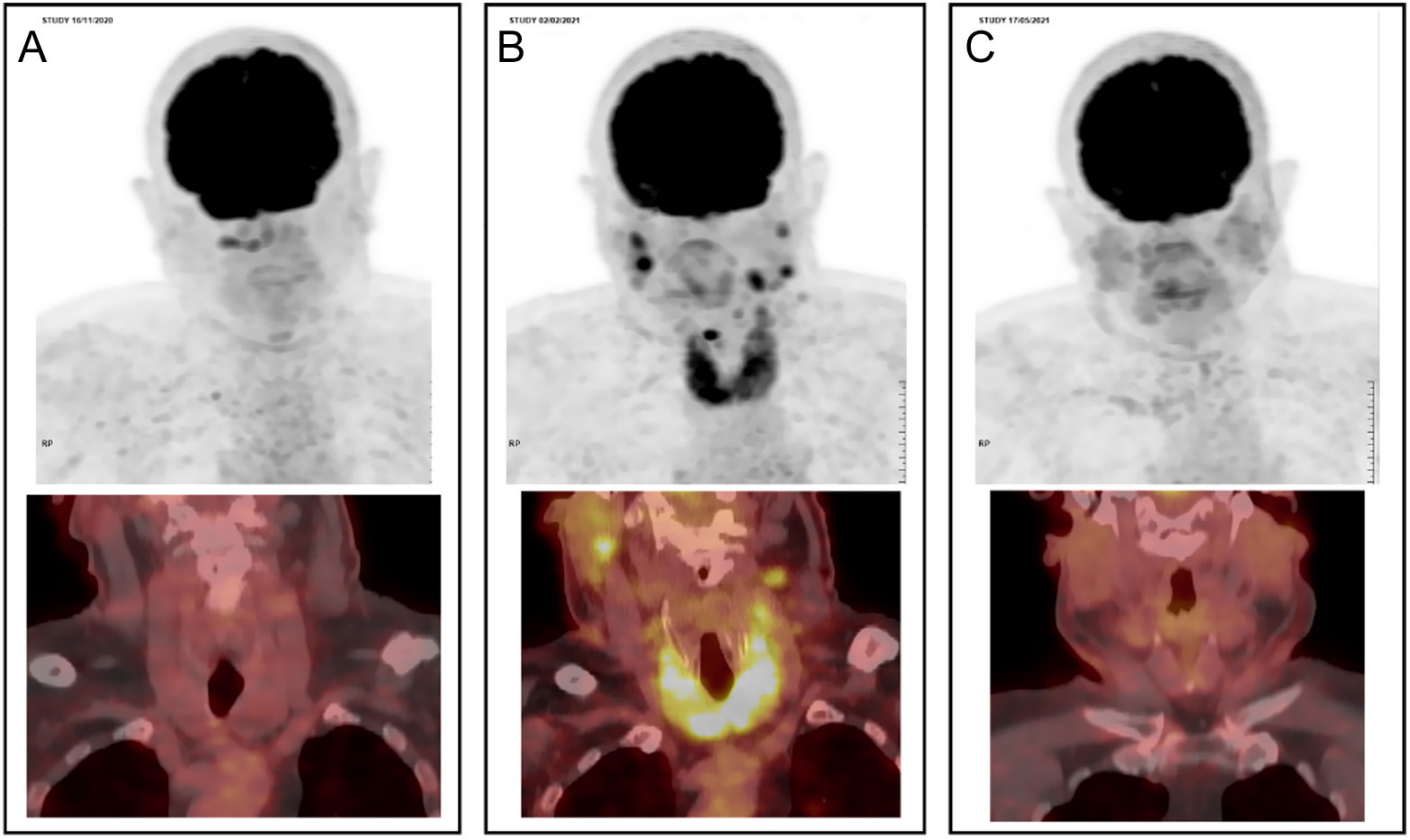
(A) ^18^F-FDG PET/CT before ICI initiation (November 2020) showed absent thyroid hypermetabolism. (B) 2 months after ICI initiation (February 2021), an intense and diffuse ^18^F-FDG uptake was observed in the thyroid gland (SUV_max_ 9.5). (C) ^18^F-FDG PET/CT performed in May 2021, after ICI withdrawal, showed regression of thyroid ^18^F-FDG uptake and thyroid atrophy.
